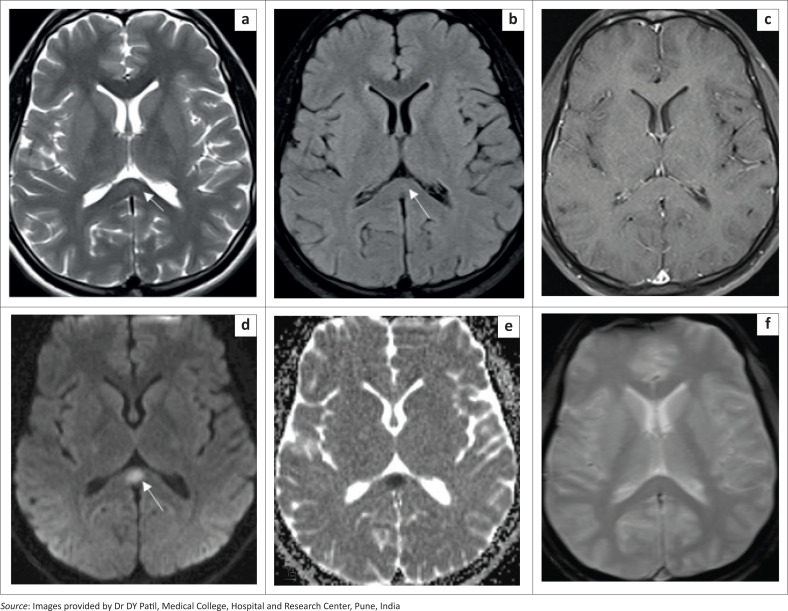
[Case 3]: Brain MRI of a 25-year-old male diagnosed with dengue fever showing a non-enhancing oval shaped T2 (a) and FLAIR (b) hyperintense lesion (white arrow) in the splenium of the corpus callosum. The lesion demonstrated no enhancement (c) and bright signal at DWI (d) and corresponding low values on apparent diffusion coefficient (e) with no blooming on the GRE images (f).
